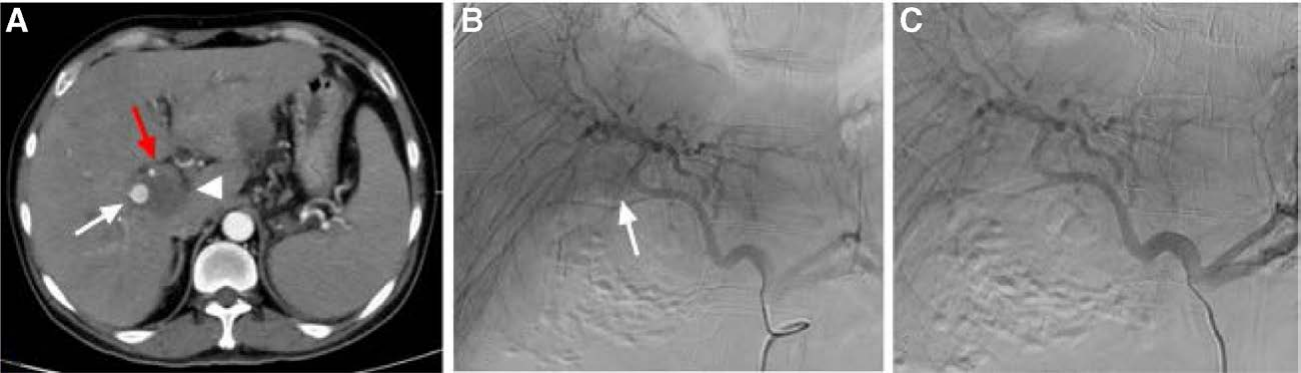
(A) Abdominal CT scan showed the presence of a right HAP (white arrow), a hematoma locating in hepatic hilum (white arrowhead) and slightly dilated bile duct (red arrow). (B)Celiac angiography confirmed the right HAP (white arrow) in the right hepatic artery, but the feeding branch of the right hepatic artery was not clear. (C) Another celiac angiography showed spontaneous disappearance of the right HAP. CT = computed tomography, HAP = hepatic artery pseudoaneurysm.
